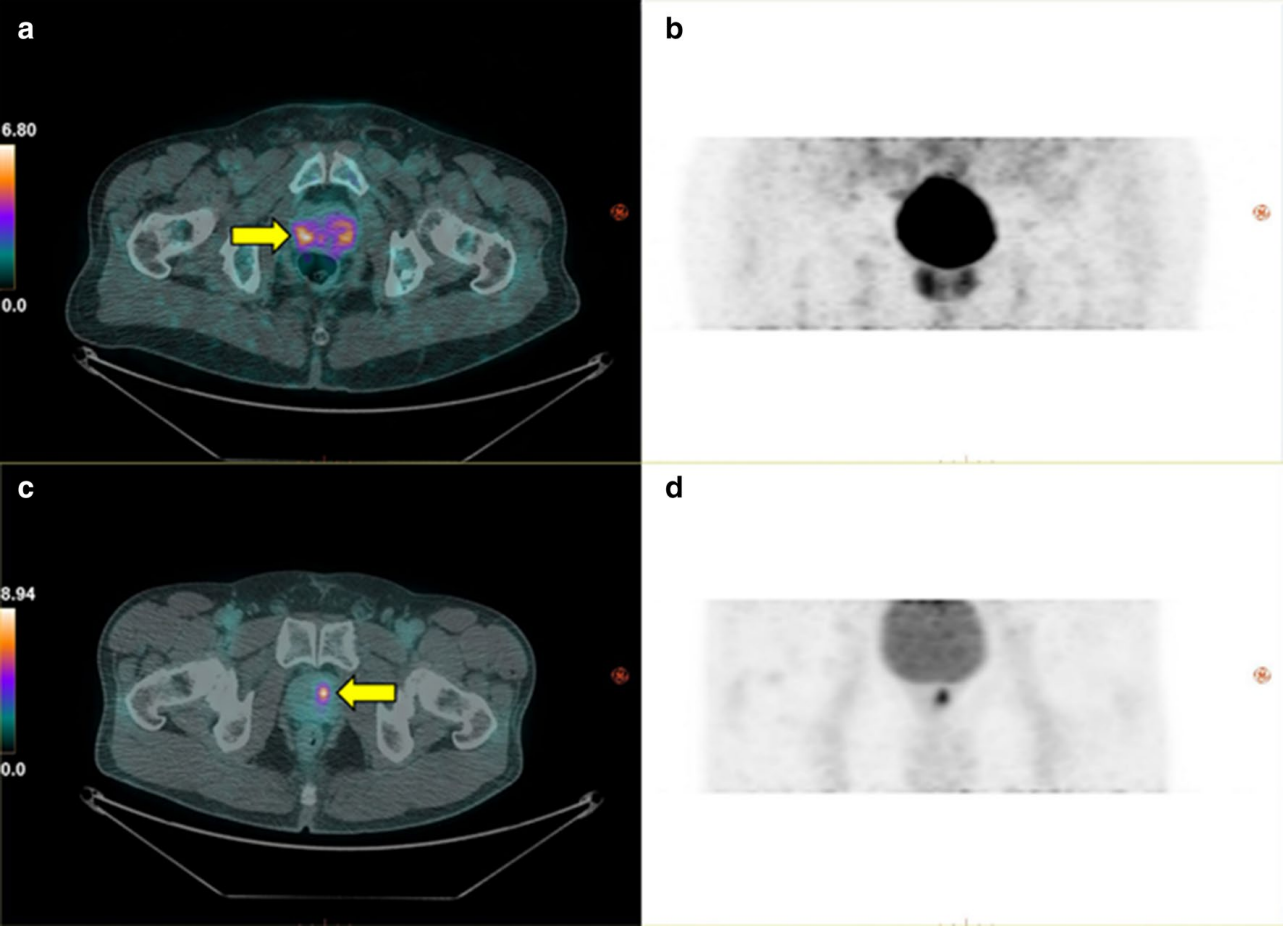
PET/CT images of the same patients as Fig. 2 showing maximum intensity projection of [^18^F]FCH PET SUV map in an axial view superimposed on CT (a) and in a coronal view by itself (b); the same two views for a [^18^F]DCFPyL study on another patient are shown in (c) and (d). The colour bar shows the scale of SUV. Localization of DIL (yellow arrow) with PET in both patients agreed with pre-operative prostate sextant biopsy. [^18^F]DCFPyL SUV map shows superior tumour contrast relative to benign prostatic tissue and better localization of DIL than [^18^F]FCH. PET, positron emission tomography; CT, computed tomography; FCH, fluorocholine; SUV, standardized uptake value; DCFPyL, 2-(3-{1-carboxy-5-[(6-[^18^F]fluoro-pyridine-3-carbonyl)-amino]-pentyl}-ureido)-pentanedioic acid; DIL, dominant intraprostatic lesion
